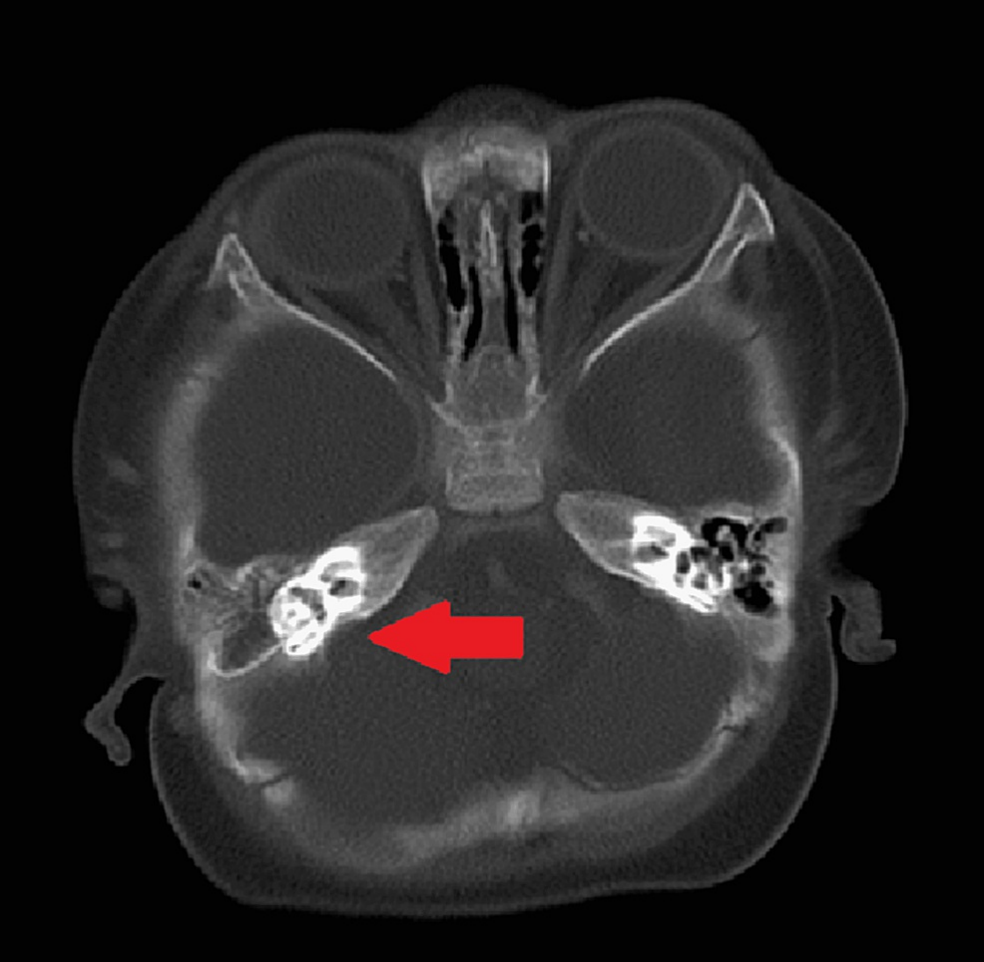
CECT brain axial view (bone window) showing fluid in the right mastoid air cells and middle ear cavity. CECT: contrast-enhanced computed tomography
